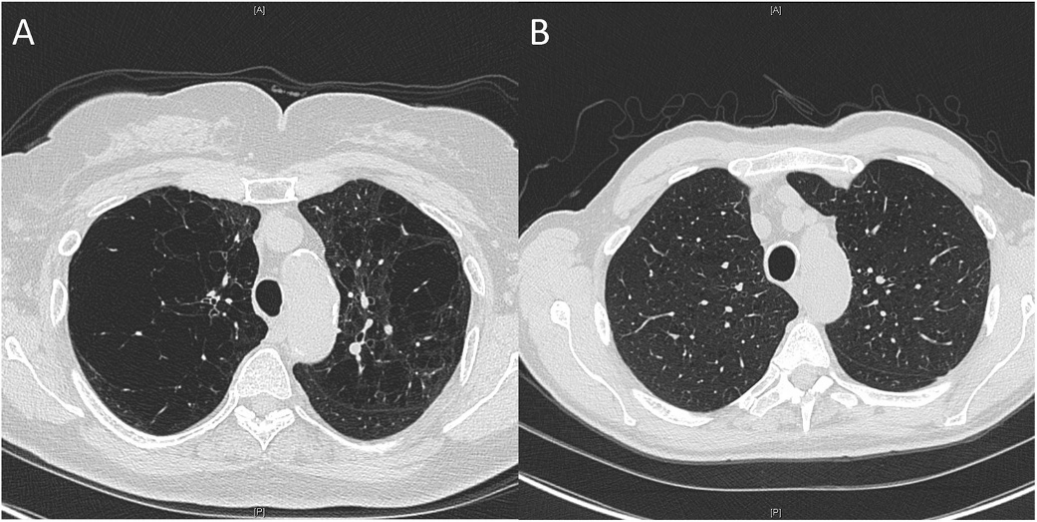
Chest CT scans from COPDGene subjects demonstrating emphysema-predominant and non-emphysematous COPD. (A) Emphysema-predominant: FEV_1_ 55.8% predicted, 29.0% emphysema. (B) Non-emphysematous: FEV_1_ 55.3% predicted, 4.2% emphysema.
